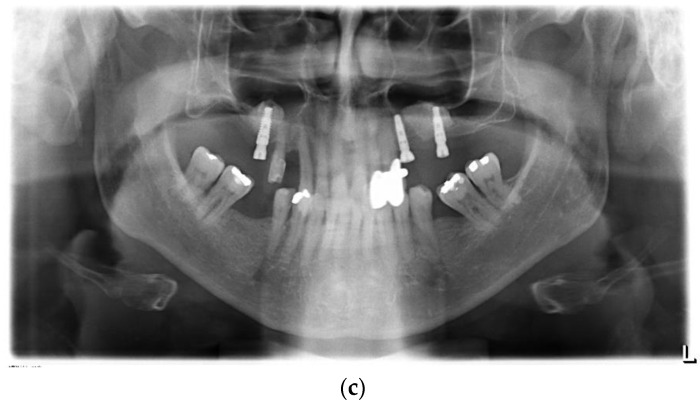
Radiographical outcome (a) Sagittal plane of the left maxillary sinus directly after augmentation. An air-fluid-level of blood is visible after a small perforation of the Schneiderian membrane. The scaffold is tightly fixed to the host bone by the screw. (b) Sagittal plane of the left maxillary sinus prior to implant placement. The scaffold is showing sufficient osseointegration. The bone lid is visible cranial of the scaffold. No sign of inflammation or mucosal swelling is detectable in the sinus. (c) Panoramic radiograph after implant uncovering. A sufficient osseointegration of the scaffolds and the dental implants without any signs of peri-implant osteolysis is obvious. No sign of inflammation of the maxillary sinuses is detectable.
